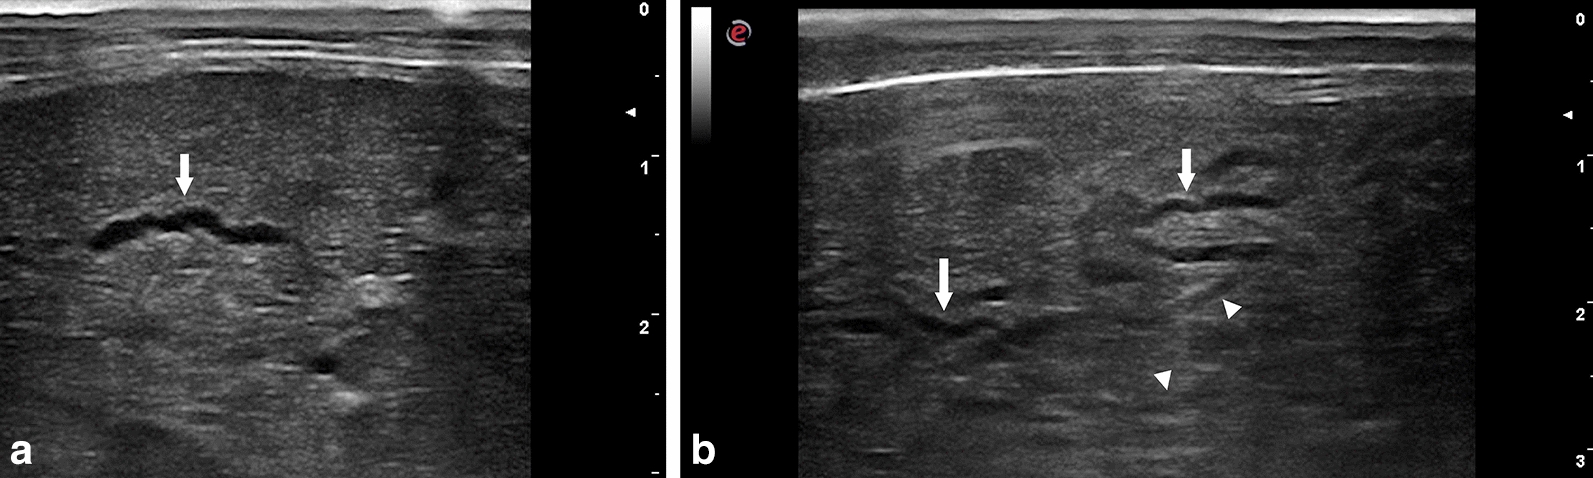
Ultrasonographic appearance of hepatic migratory tracts produced by *cysticercus tenuicollis*. a Ultrasonographic aspect of a hepatic intraparenchymal anechoic tract (arrow). b Ultrasonographic aspect of several hepatic intraparenchymal anechoic (arrow) and hypoechoic (head arrow) tracts
